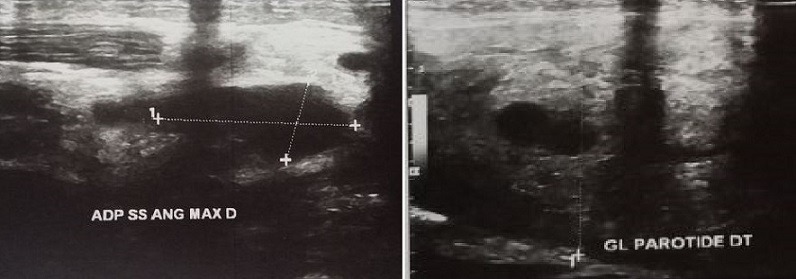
Cervical ultrasound showing inflammatory angulomaxillary adenopathy (A) and parotitis on the right (B)
